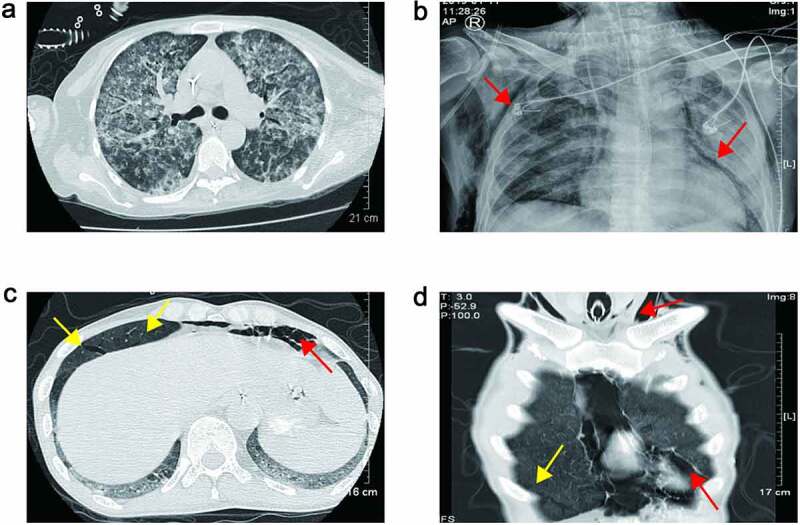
(a) Chest CT scan revealed bilateral diffuse infiltration indicated pulmonary hemorrhage of Patient 3; (b) chest plain radiograph showed pneumomediastinum and subcutaneous emphysema of Patient 5 (red arrow). (c) and (d) Chest CT scan showed pneumomediastinum, subcutaneous emphysema (red arrow) and parenchymal tears of Patient 7 (yellow arrow)
